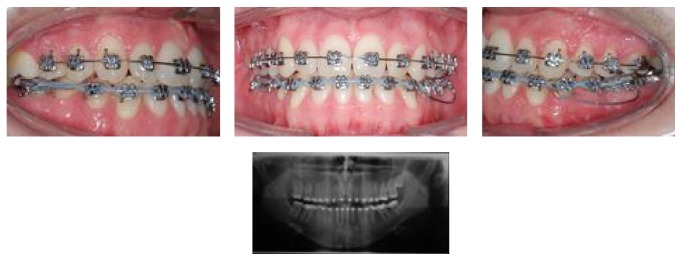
Intraoral views of treatment and panoramic radiograph. Bonding tooth 38, cantilever applied to upright tooth 37, and elastic chain to close the remaining spaces. Panoramic radiograph showing uprighted good position of tooth 38.
